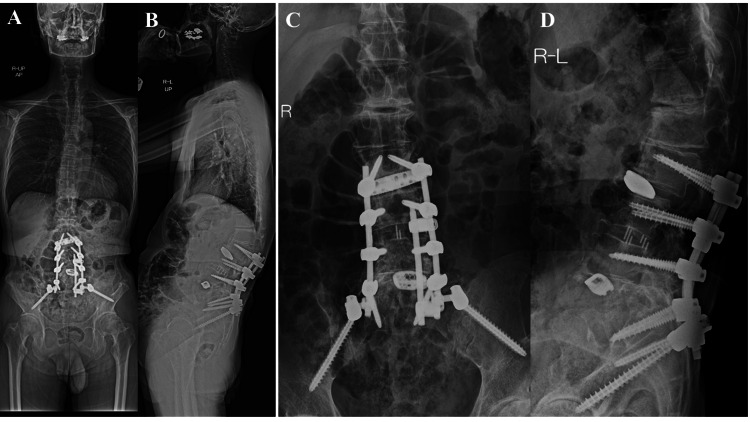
Postoperative plain X-ray imaging of the spine and hip at two years (A) Postoperative plain X-ray of the whole spine (anterior-posterior view). (B) Postoperative plain X-ray of the whole spine (lateral view) shows improved lumbosacral alignment. (C) Postoperative plain X-ray of the lumbar spine (anterior-posterior view). (D) Postoperative plain X-ray of the lumbar spine (lateral view) displays LLIF at the L2/L3 and L3/L4 levels using an LLIF cage, and posterior spinal fusion with PSO. LLIF: lateral lumbar interbody fusion, PSO: pedicle subtraction osteotomy
